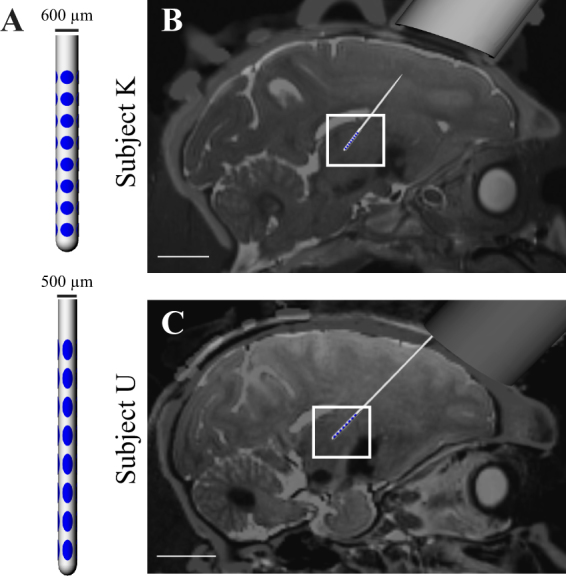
DBS Array Implants. (A) DBS arrays implantated in the cerebellar-receiving area of motor thalamus. (B,C) Co-registration of pre-operative T2-weighted MRI and post-implant CT images showing the final DBS array position and orientation in each subject. 1-cm scale bars are shown in (B,C).
